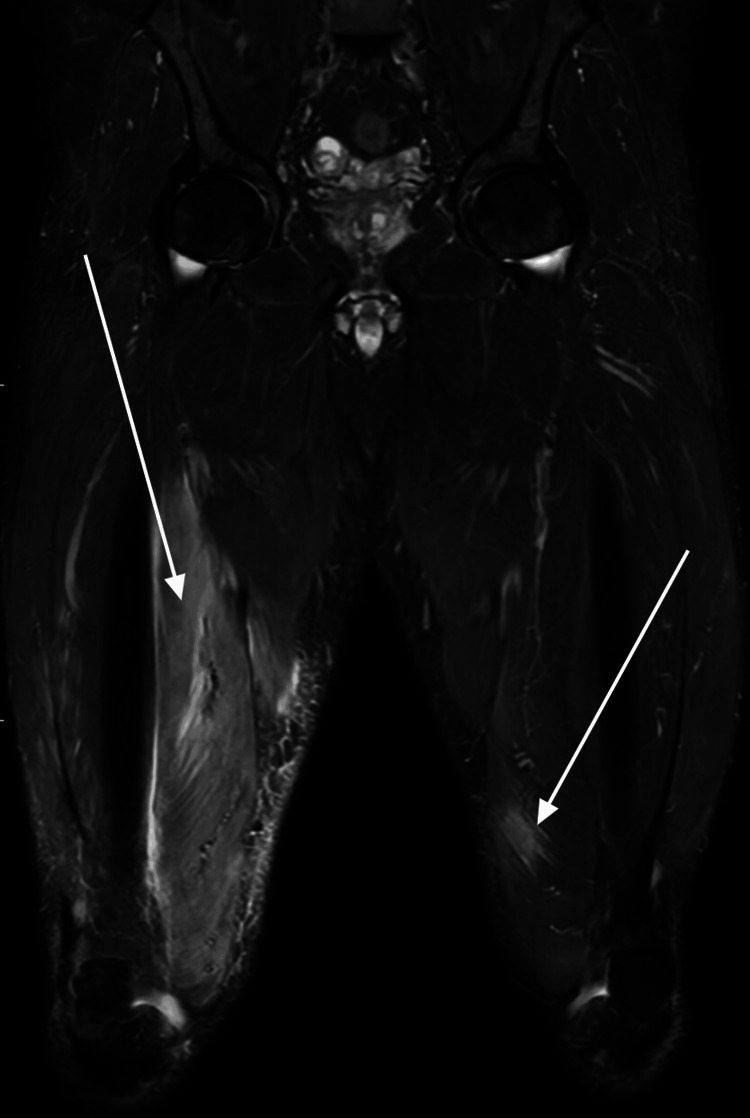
T2-weighted coronal MRI of both thighs showing improvement of left thigh muscle edema and worsening of right thigh muscle edema (white arrows).
